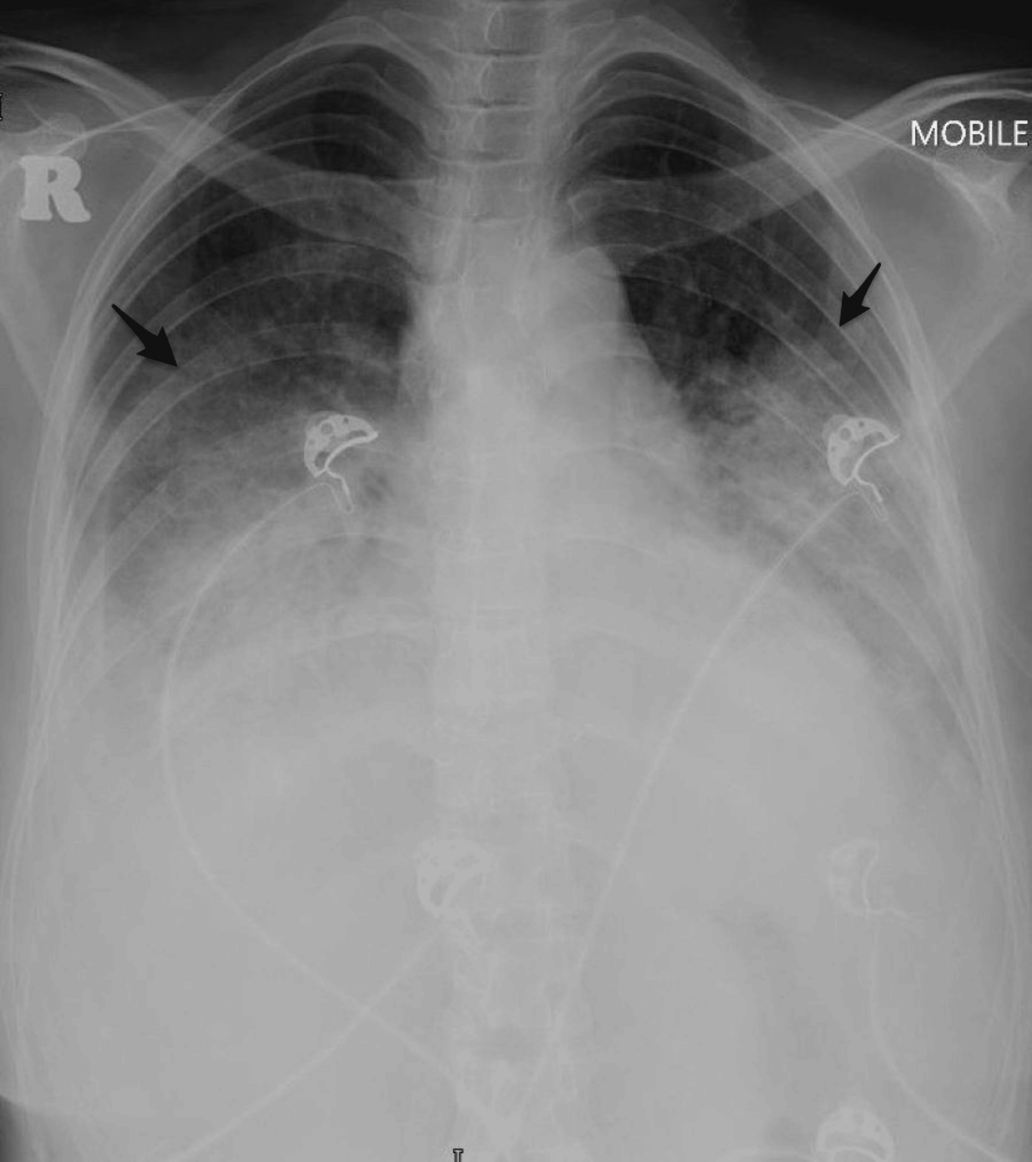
Chest X-ray on 27.8.2024 (day 10 of admission) Repeated chest radiograph at day 10 of admission showing bilateral interstitial-alveolar opacities in the perihilar regions (black arrows), in keeping with pulmonary congestion.
